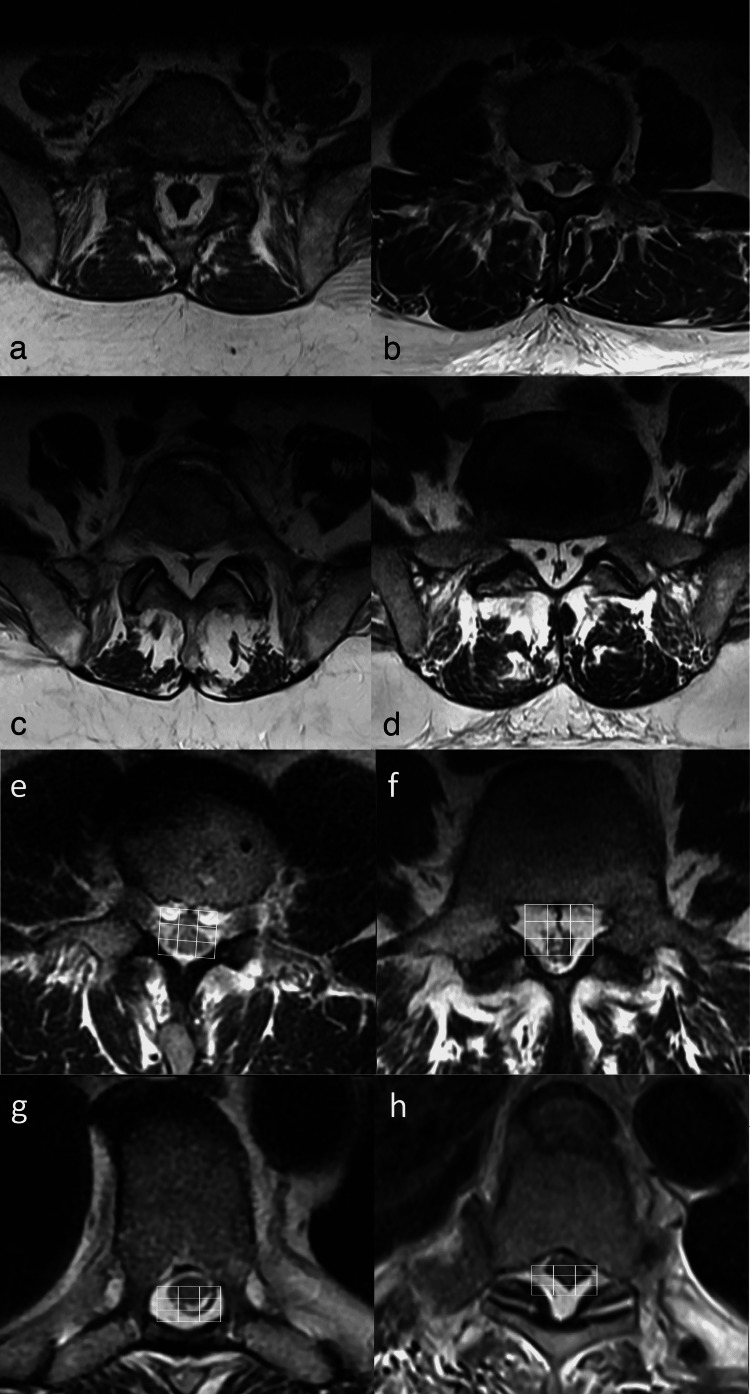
a-d: Axial T2-weighted MR images demonstrating different thecal sac morphologies in Manjila Type III lumbar SEL a) polygonal, b) stellate, c) Y-shaped and d) nearly obliterated thecal sac. Oftentimes, the radiological severity does not translate to the clinical severity of radiculomyelopathy or neurogenic claudication. e-f: Axial T2-weighted MR images demonstrating a novel 3x3 grid method in e) mild type Manjila Type I (ventral) and f) moderate Manjila Type I (ventral) SEL cases. g-h: depict a thoracic SEL Manjila Type II (dorsal) variant, where a 3x3 grid can be readily applied, as against a 3+1 or trifoliate grid required at the L5-S1 level. SEL: spinal epidural lipomatosis Source: Authors of the current article
